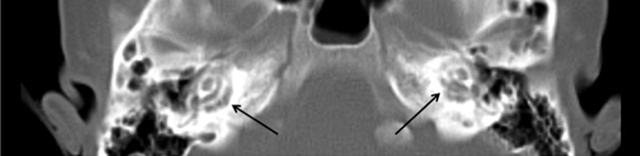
Axial CT-scan shows bilateral lucencies in the pericochlear bony otic capsule.
